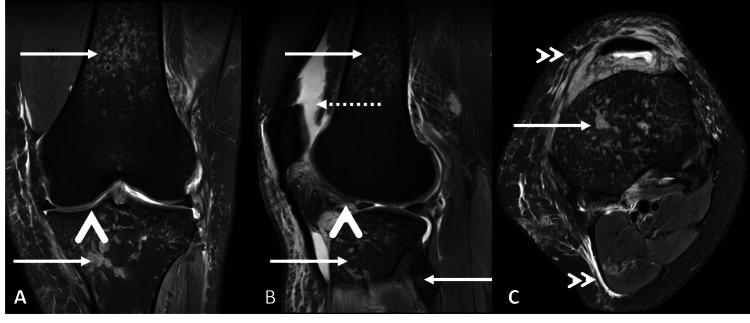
MRI of the left knee joint A, B, & C: PDFS sequence in coronal (A), sagittal (B), and axial (C) planes of the knee joint and distal thigh. Geographical areas of altered marrow signal intensities are noted in the distal shaft of the femur, proximal tibia, and fibula (solid white arrow). Moderate knee joint effusion noted extending into the suprapatellar recess (dotted white arrow). Severe reduction in the joint space with mild synovial thickening is noted (arrowhead). Extensive soft tissue edema surrounding the knee joint and distal thigh (double arrowhead). PDFS: proton density fat-saturated
